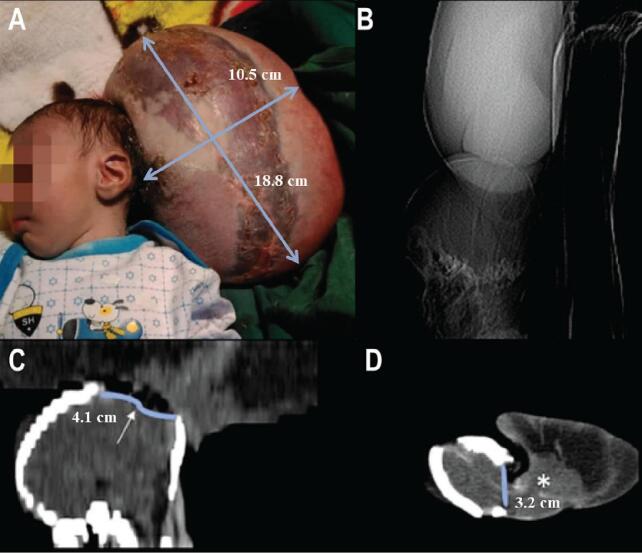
(A) Preoperative photograph showing a giant occipital encephalocele. (B) A preoperative plain radiograph shows a huge soft tissue mass. (C, D) Sagittal and coronal sections of the preoperative non-enhanced brain computed tomography scan. A bone defect is seen (blue line), with huge herniated meninges and brain tissues.
